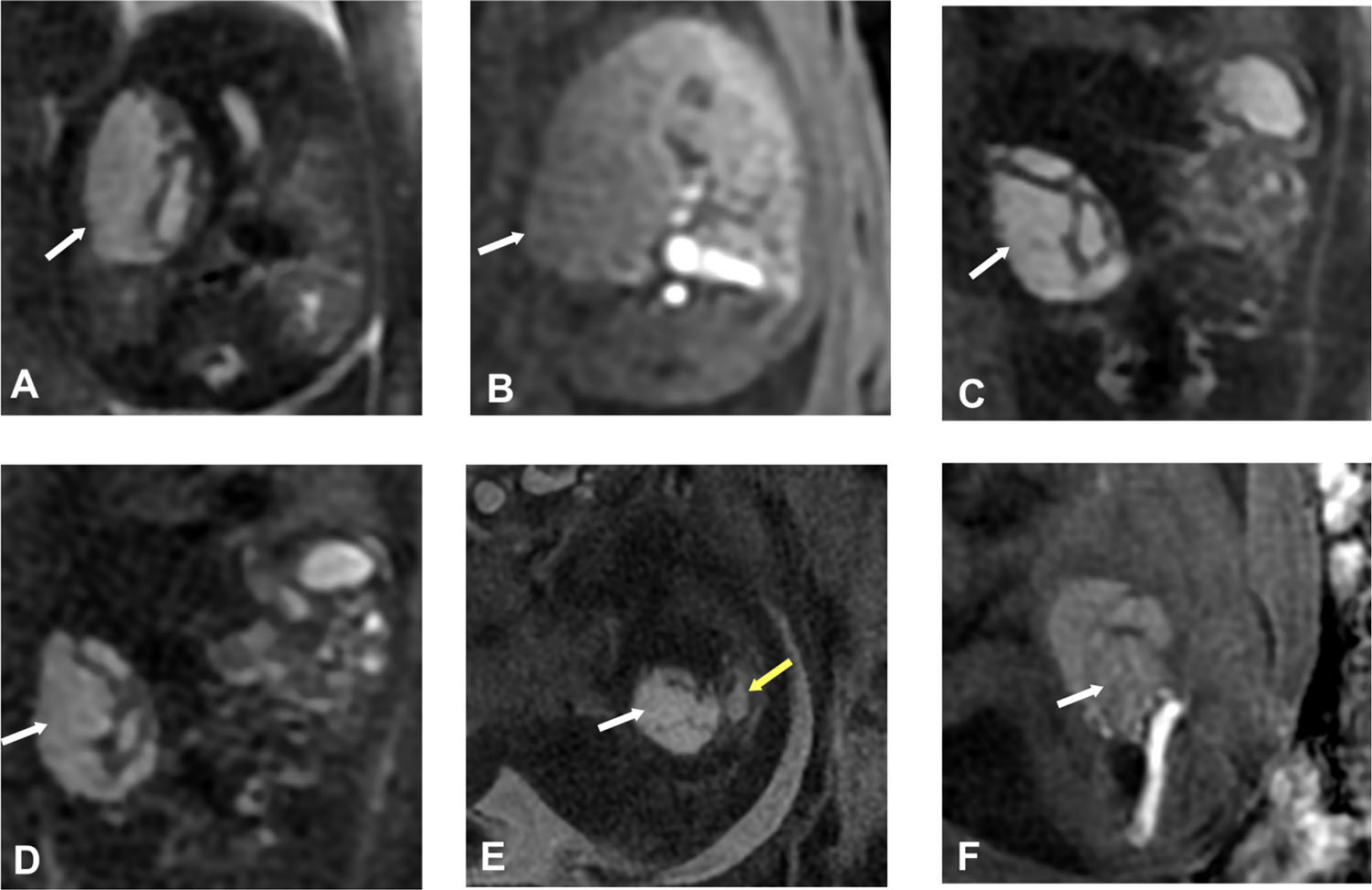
Hepatic mesenchymal hamartoma. Fetal ultrasound: septated hepatic cyst. Gestational age 21 weeks and 1 day. A Axial SS FSE T2. B Axial 3D LAVA. C, D Coronal SS FSE T2. E Sagittal FIESTA. F Sagittal 3D LAVA. Cystic mass (white arrows) in the right hepatic lobe, with septations inside, exophytic, isointense on T1 and hyperintense on T2, causing mild right renal ectasia (yellow arrow), suggesting mesenchymal hamartoma
